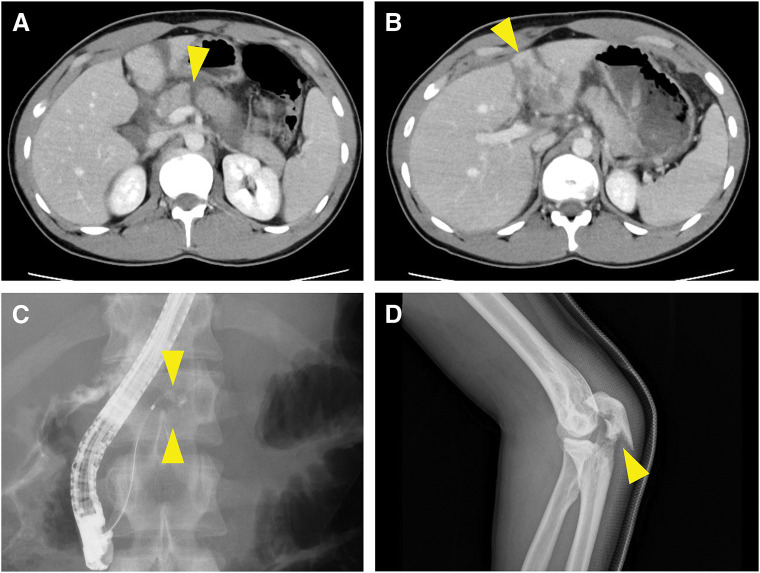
Images before surgical intervention in Case 1. (A) A contrast-enhanced CT scan after admission to the previous hospital revealing a laceration of the pancreatic body (arrowhead). (B) A contrast-enhanced CT scan after admission to the previous hospital showing a laceration and compression of the liver (arrowhead). (C) ERP showing the leakage of contrast agents from the MPD. (D) X-ray image showing a fracture of the left olecranon (arrowhead). CT, computed tomography; ERP, endoscopic retrograde pancreatography; MPD, main pancreatic duct
